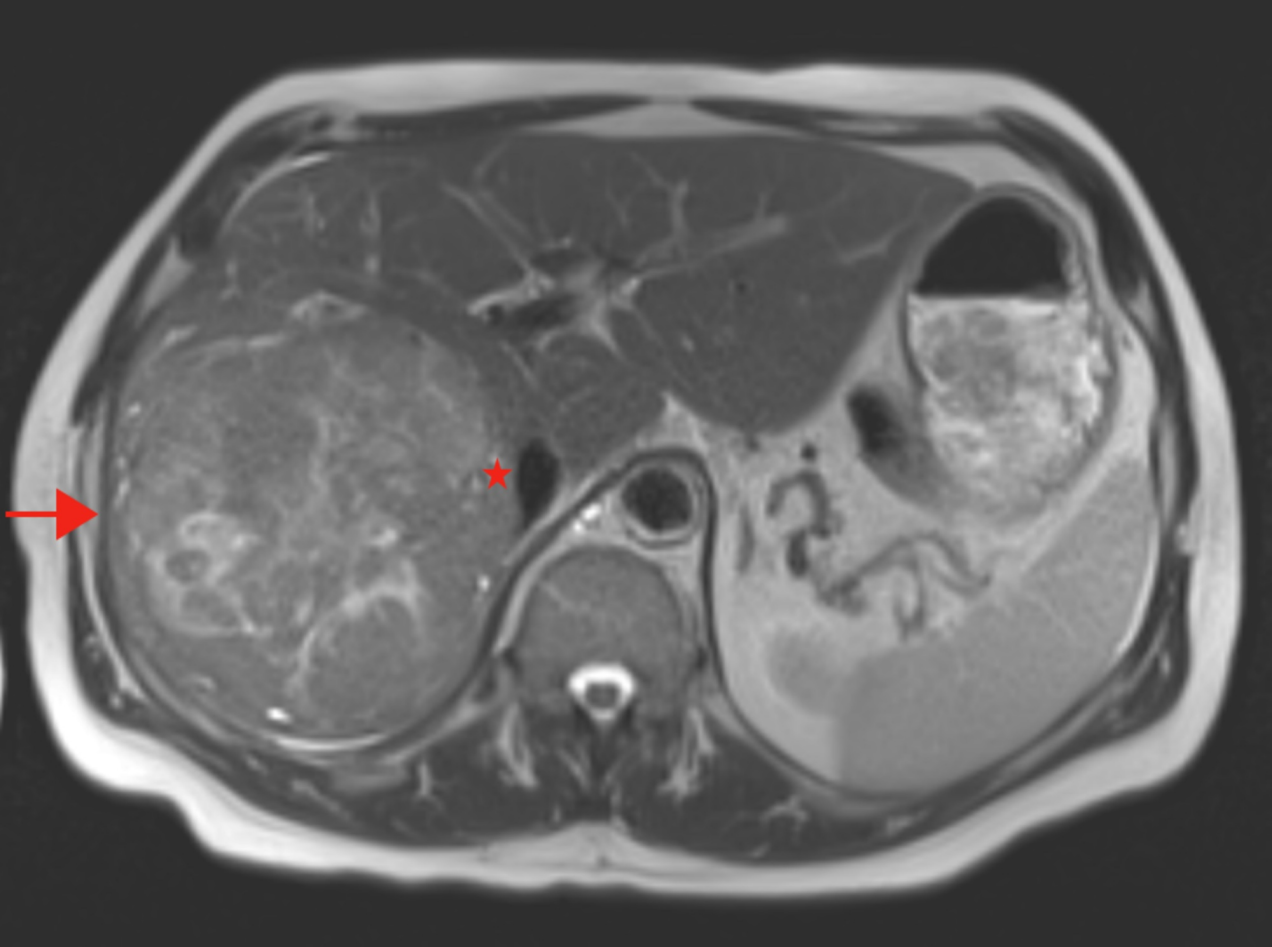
Presentation MRI of the abdomen A large heterogeneous mass in the right lobe of the liver is seen (arrow). Mild extension into the lateral wall of the intrahepatic inferior vena cava is also demonstrated (star).
